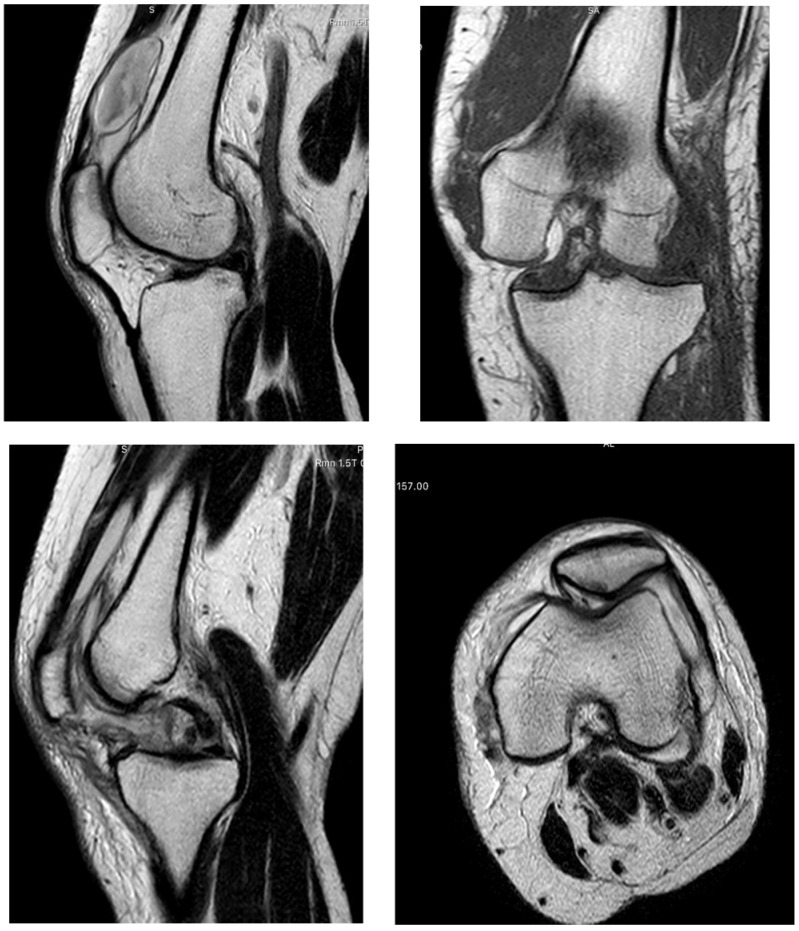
MRI showing extensive soft tissue swelling and effusion, knee dislocation with intra-articular medial capsule subluxation, complete rupture of the ACL, PCL, and MCL, and lateral subluxation of the patella.
